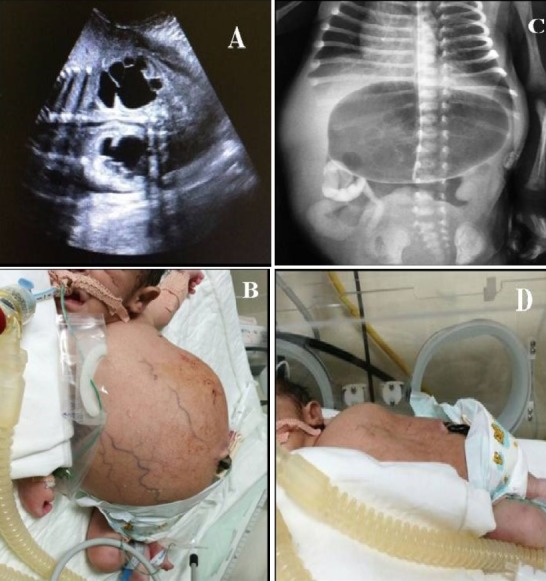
A) antenatal ultrasonography shows fetal bilateral hydronephrosis; B) massive distended abdomen secondary to distended urinary bladder; C) abdominal X ray shows dilated stomach; D) after vesicostomy, reduced abdominal distension
